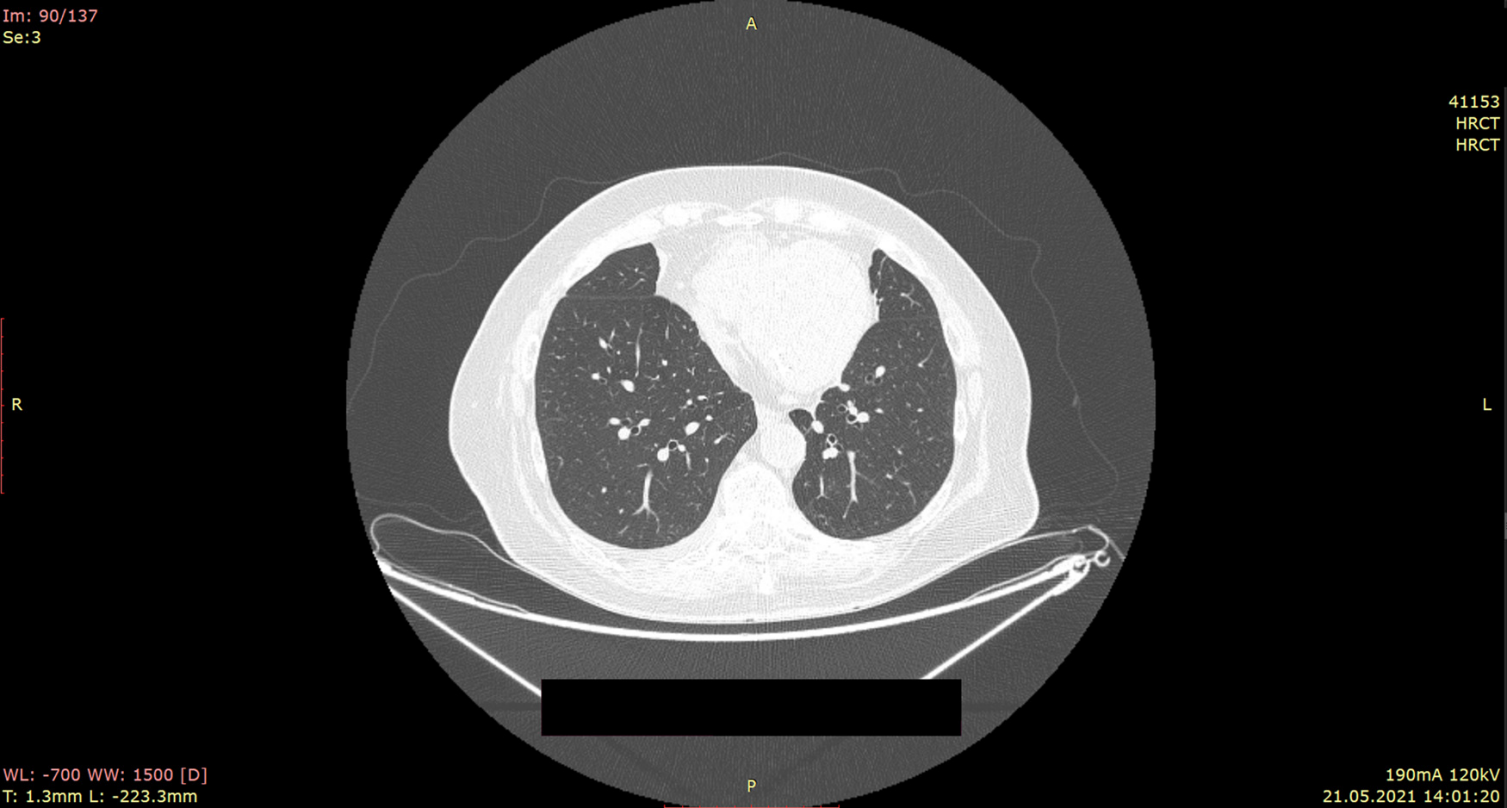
HRCT scans after antifungal treatment: regression of changes visible on admission is described.
